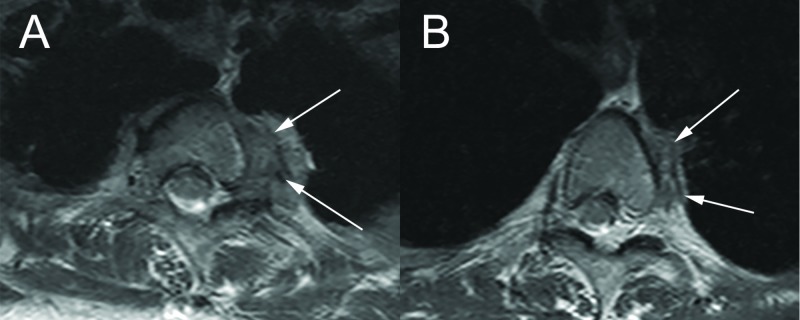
Axial MRI of T2 and T3 Thoracic Vertebrae Axial T2W magnetic resonance (MR) images of the thoracic spine. On the left of the vertebral bodies (A: T2 vertebral body. B: T3 vertebral body), the tumor mass is delineated by white arrows. MRI: magnetic resonance imaging
